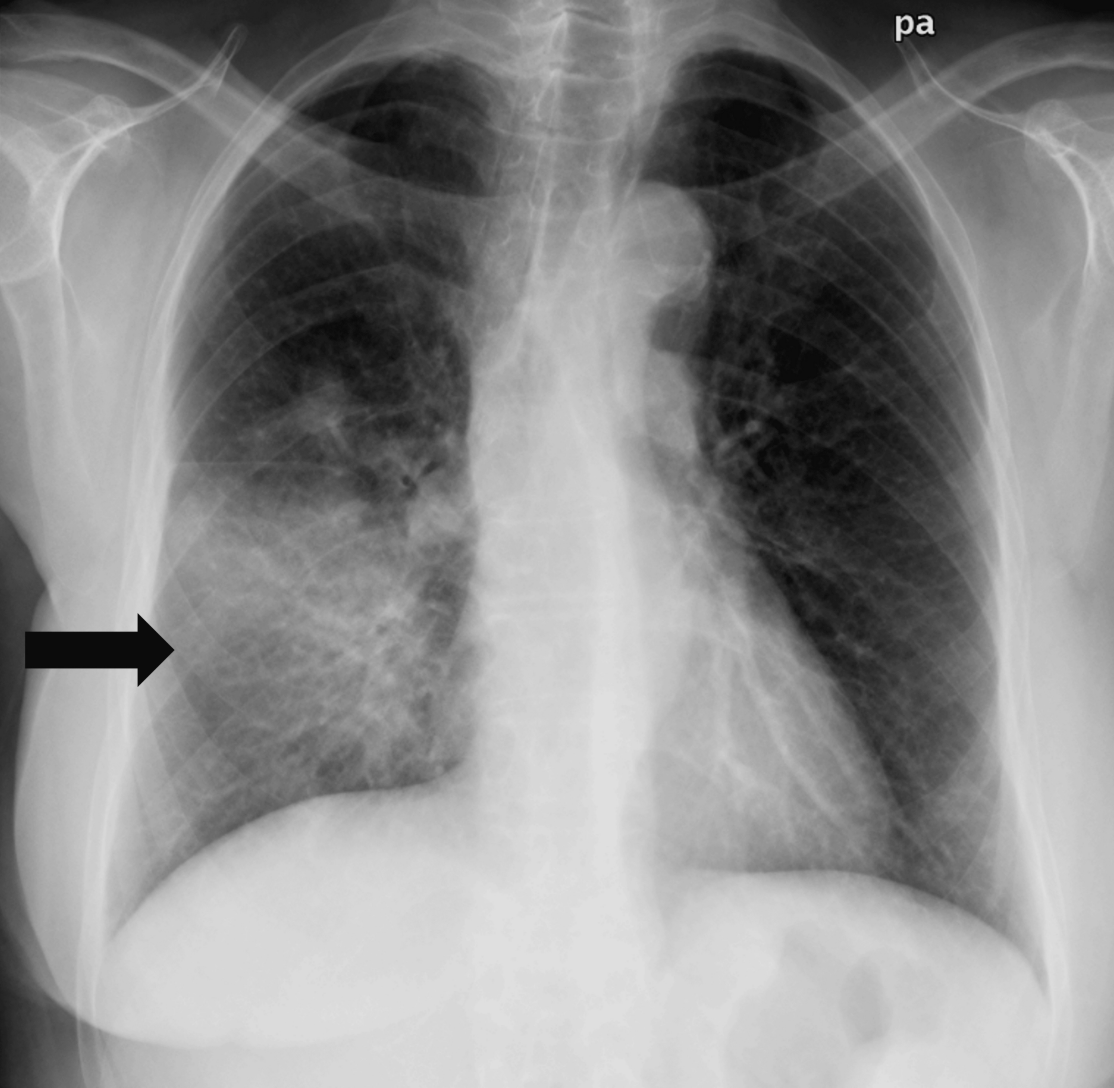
Initial thoracic X-ray showing right lung opacities (black arrow).
